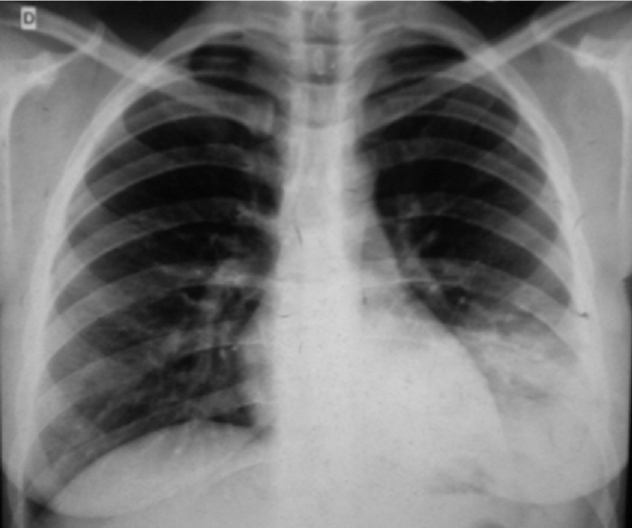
Radiographie thoracique de face: opacité alvéolaire basithoracique gauche
